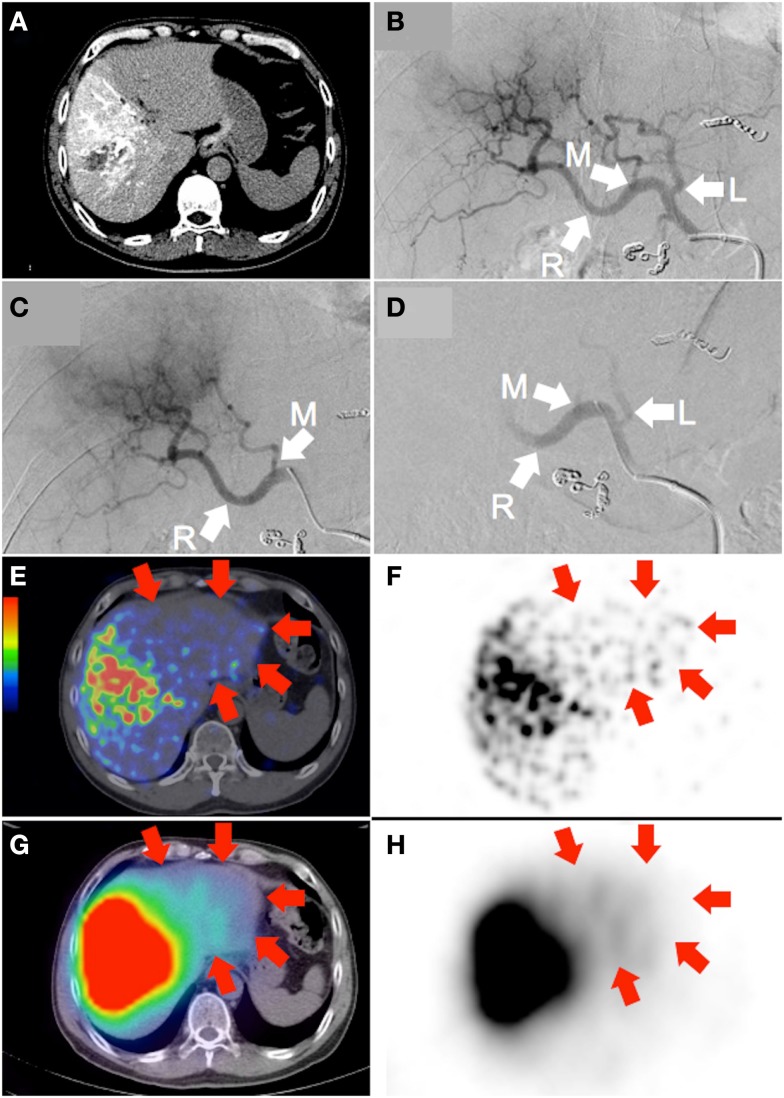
Large hepatocellular carcinoma of the right lobe (actual tumor not well depicted). (A) Catheter-directed CT angiogram of the right hepatic artery, proximal to the origin of the middle hepatic artery, delineates the target arterial territory. (B) Digital subtraction angiography (DSA) of the proper hepatic artery demonstrates the hepatic arterial tree. The liver is supplied by the left hepatic artery (“L”; branch of the common hepatic artery), middle hepatic artery (“M”; branch of the right hepatic artery) and right hepatic artery (“R”; continuation of the common hepatic artery). Prophylactic coil embolization of the gastroduodenal, right gastric, and accessory left gastric arteries were performed. (C) DSA of the target arterial tree with the catheter tip in the right hepatic artery, proximal to the origin of the middle hepatic artery, immediately prior to RE. (D) Moderate vascular stasis and contrast reflux into the left and proper hepatic arteries is seen on DSA immediately after RE, with no change in catheter tip position. (E,F)
